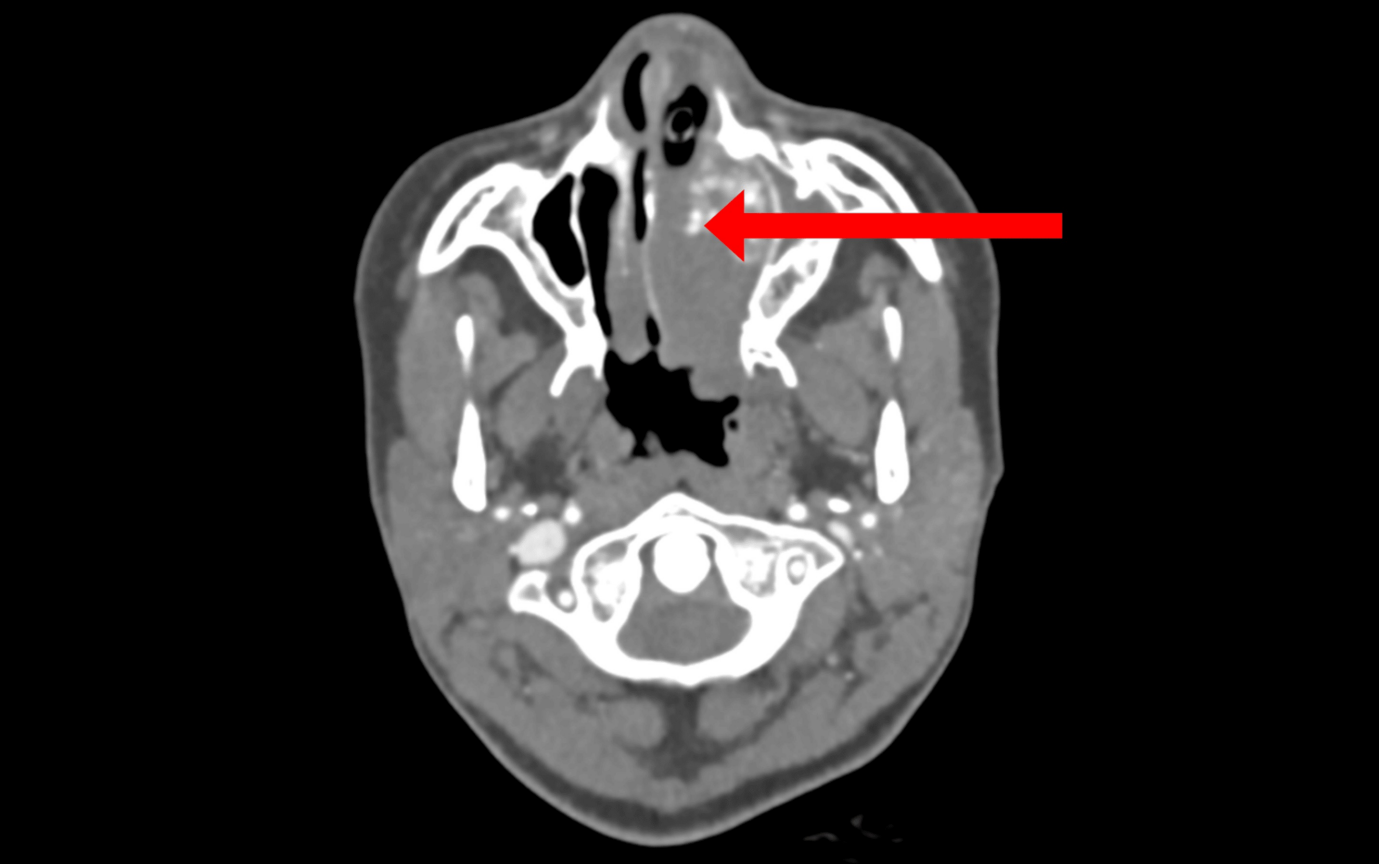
CT shows a well-circumscribed soft-tissue mass in the left nasal cavity with outward bowing of the medial wall of the maxillary sinus (red arrow).
